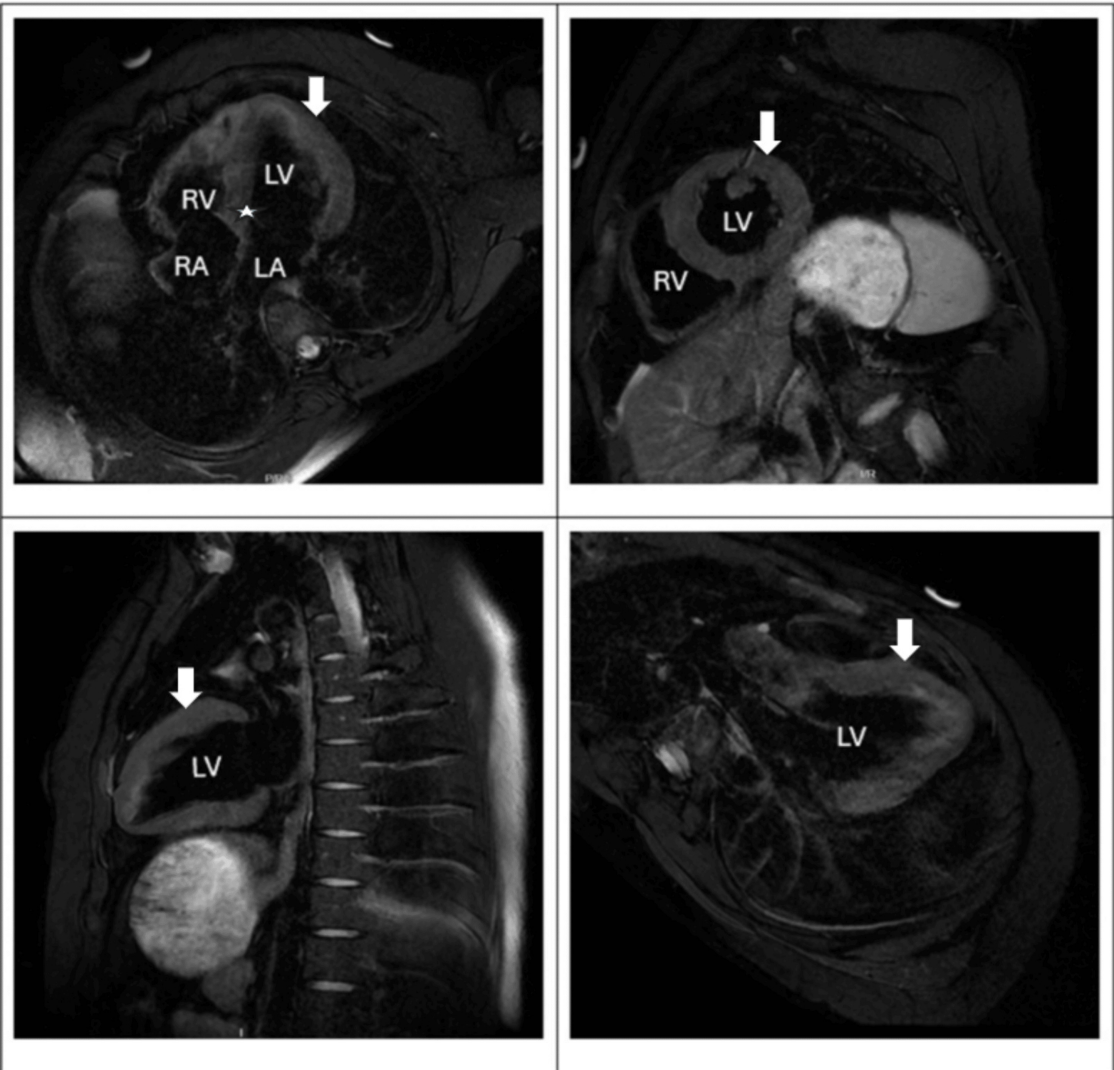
Cardiac MRI with and without contrast. Arrows indicate normal left ventricular size with normal systolic function; no abnormal late gadolinium enhancement. Asterisk indicates mild hypertrophy of the interventricular septum measuring up to 15 mm in the basal anterior septum. No evidence of left ventricular outflow tract obstruction. MRI: magnetic resonance imaging; LA: left atrium, LV: left ventricle, RA: right atrium, RV: right ventricle.
